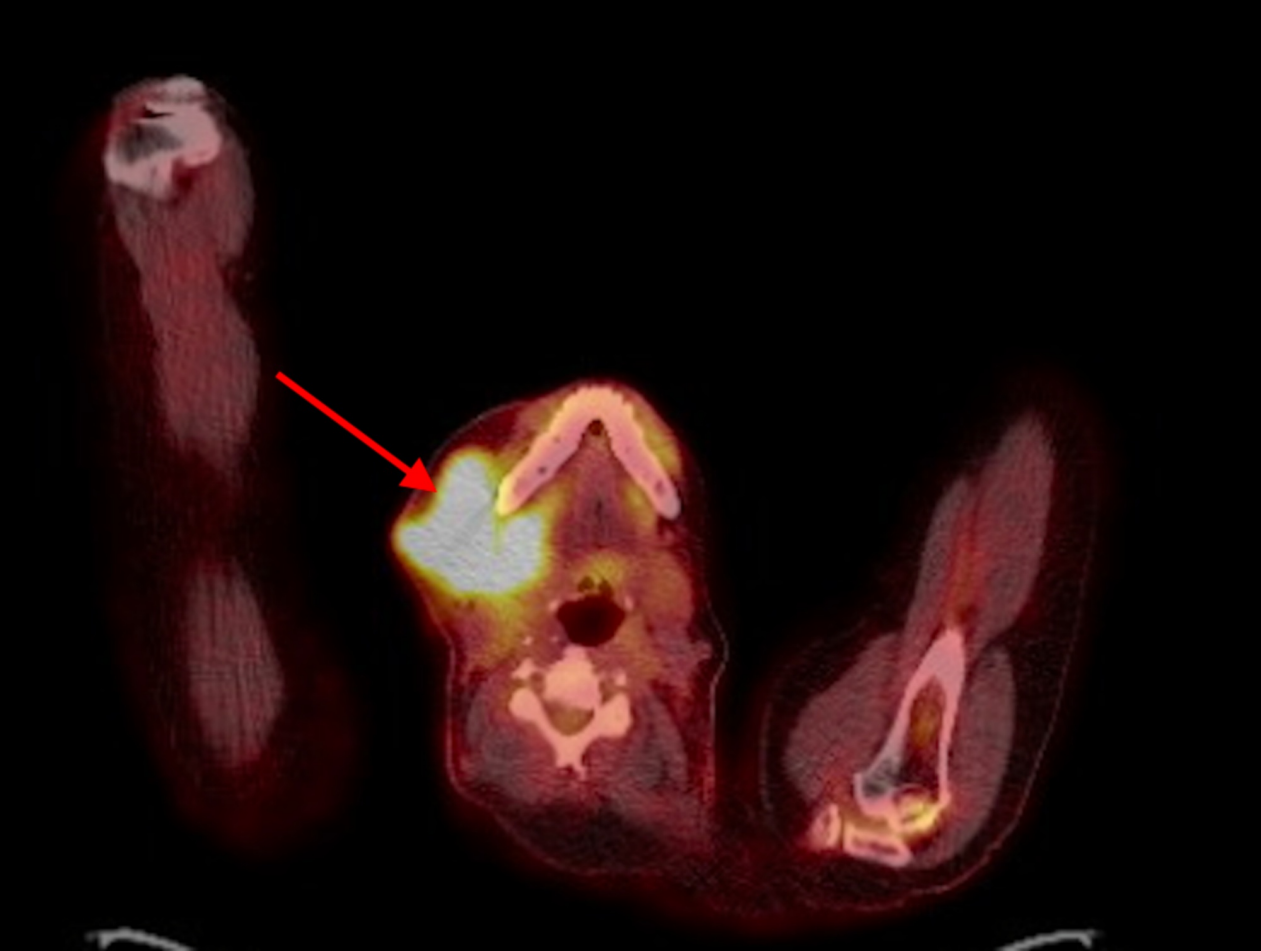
PET/CT showing the hypermetabolic right facial soft tissue mass (red arrow). PET, positron emission tomography
